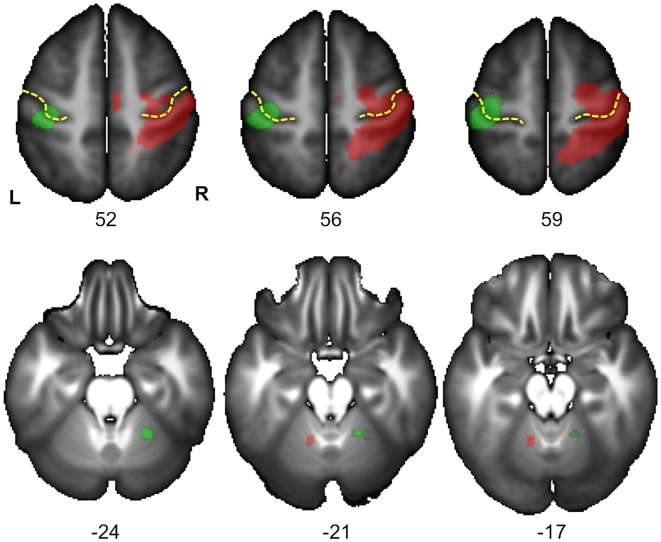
Statistical parametric maps (SPM) for the right Hand *minus* left Hand (in green) and left Hand minus right Hand (in red) contrasts in the 278 subjects who responded with their right hand. Maps are displayed at the threshold of *p* < 0.05 corrected (FWE) and superimposed on the BIL&GIN anatomical template (T1 weighted MRI averaged in 80 participants). In agreement with Figure 3 and Table 1, it displays a cluster of activation contralateral to the handedness of the presented hand in the sensorimotor cortex at the level of the hand representation including the primary motor cortex (Rolandic genu of the central sulcus in yellow). It additionally shows a cluster ipsilateral to the handedness of the presented hand in the cerebellum, in accordance with the cross-lateralized cerebral organization of motor activity (L: left, R: right, numbers correspond to the *z* coordinate of the axial slice).
